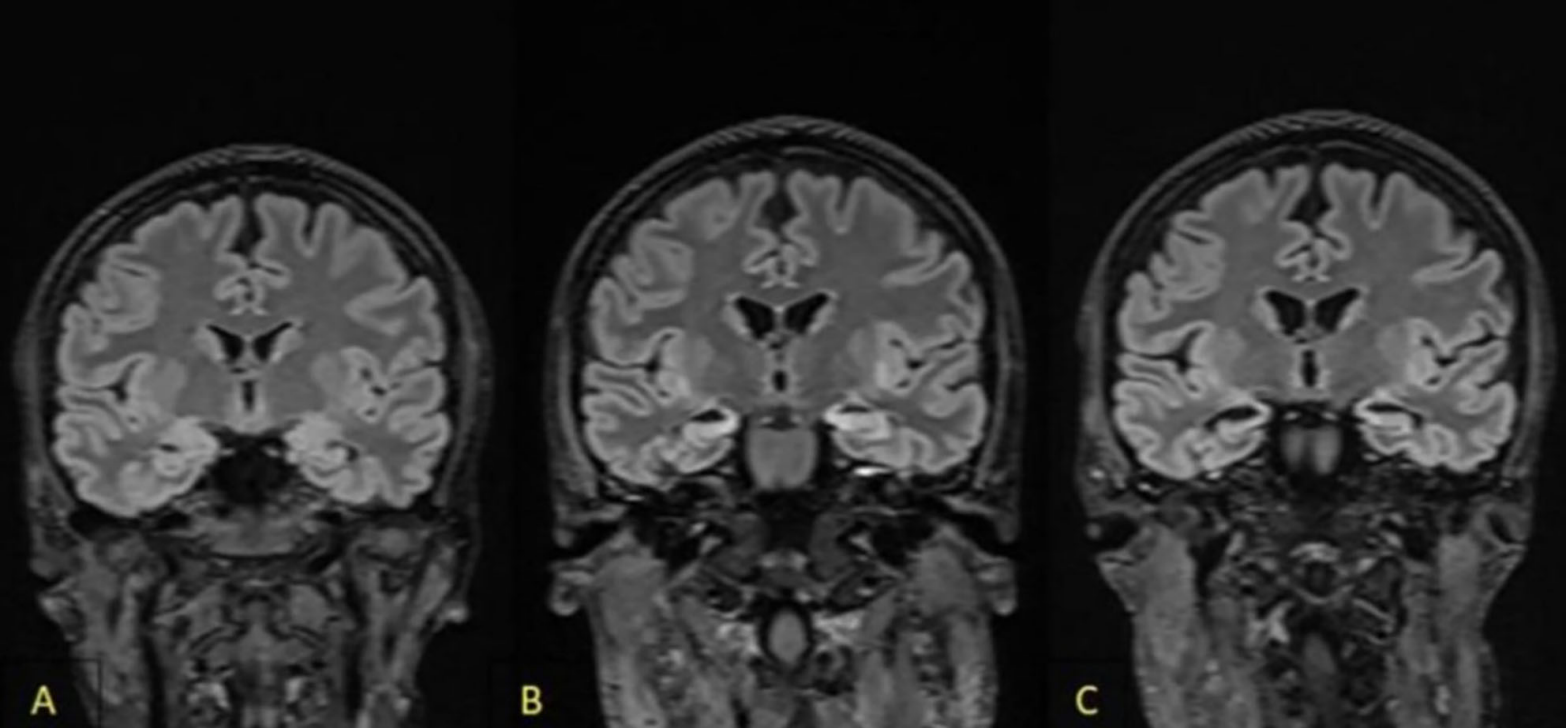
Magnetic resonance imaging (FLAIR). (A) On admission: hyperintensive bilateral lesions in both temporal lobes, mainly affected the hippocampi. (B) Hypertensive bilateral hippocampal lesions with atrophy during treatment in the intensive care unit. (C) Increased hippocampal atrophy during treatment in the psychiatry unit.
